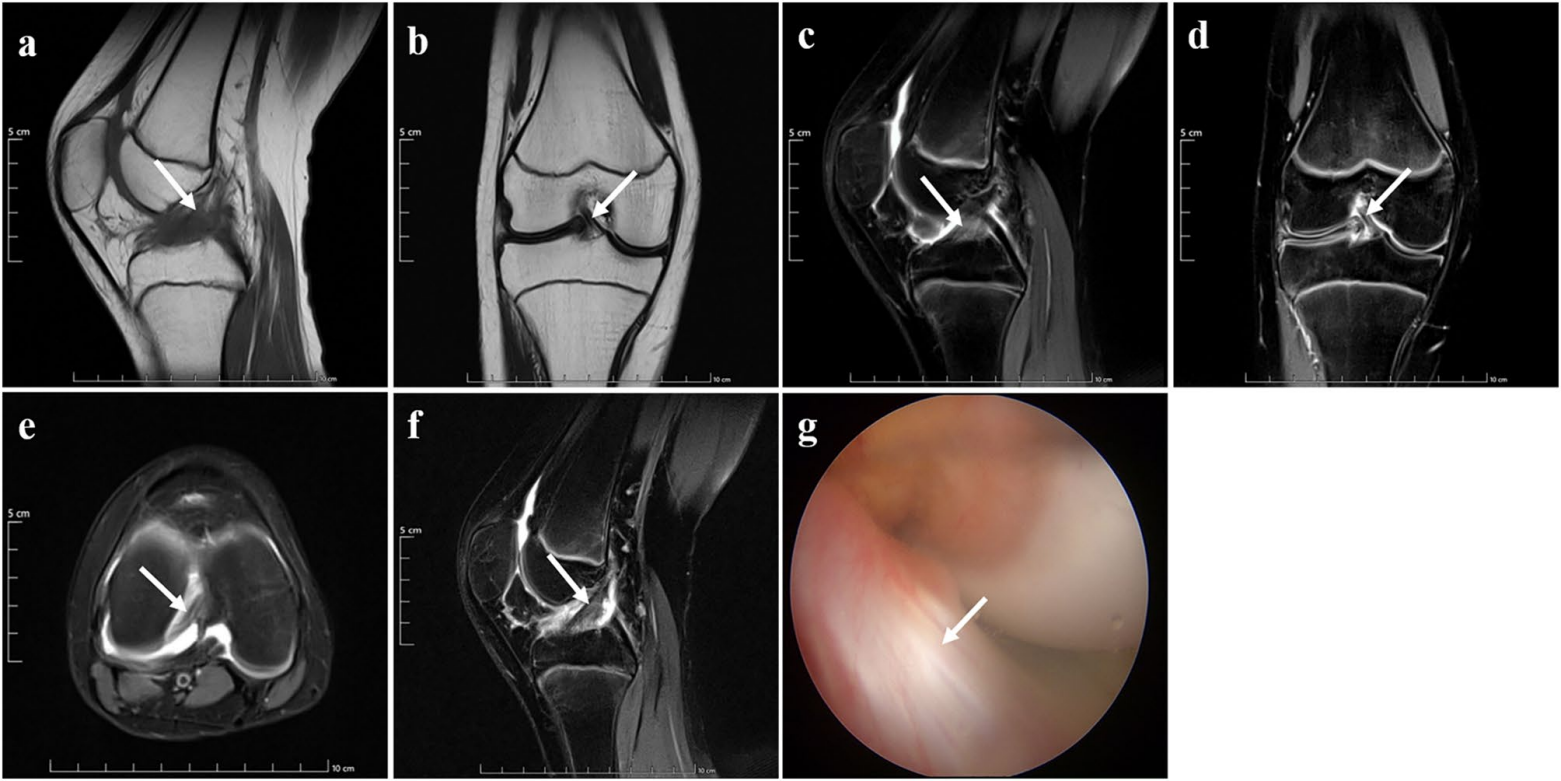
Normal ACL presence on MRI and arthroscopy of a 14-years-old female teenager. The ACL was shown on sagittal T_1_WI (a), coronal T_2_WI (b), Sagittal PDWI (c), coronal PDWI (d), and axial PDWI (e). It was tilted from the tibial to the condyle and the attachment was inserted from the condyle to the notch with low signal intensity (arrows). The ligament could be displayed completely on one level of the new modified DPP-TSO-Sag-FS-PDWI (arrow on (f)). The normal appearance of ACL was proved on arthroscopy (arrow on (g)).
